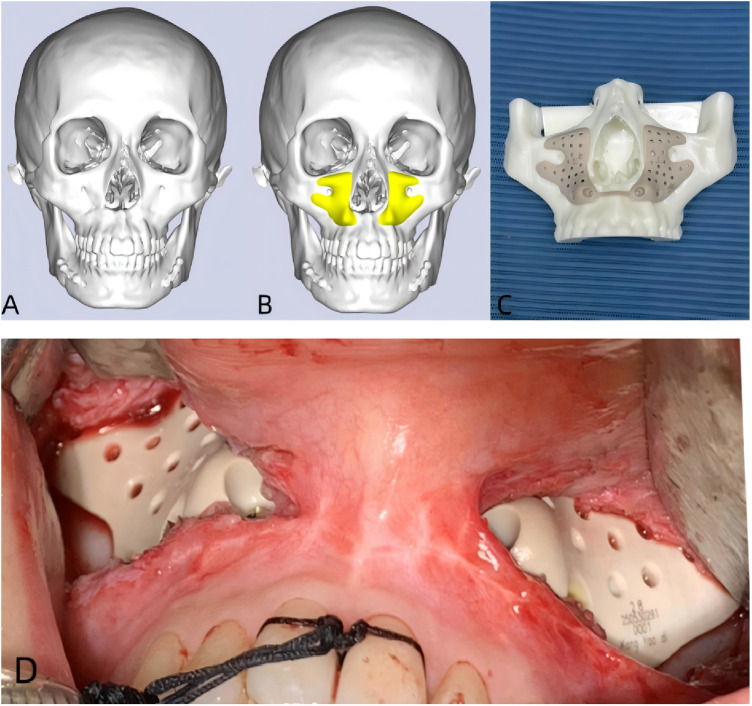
Representative case 2: preoperative defect analysis, virtual design, manufactured implant, and intraoperative fit. (A) CT showing PARA-I, PARA-U, INFRA-I, INFRA-U, and MALAR deficiency. (B) Virtual implant design based on patient goals. (C) Final CAD/CAM-manufactured IPPSI. (D) Intraoperative placement.
